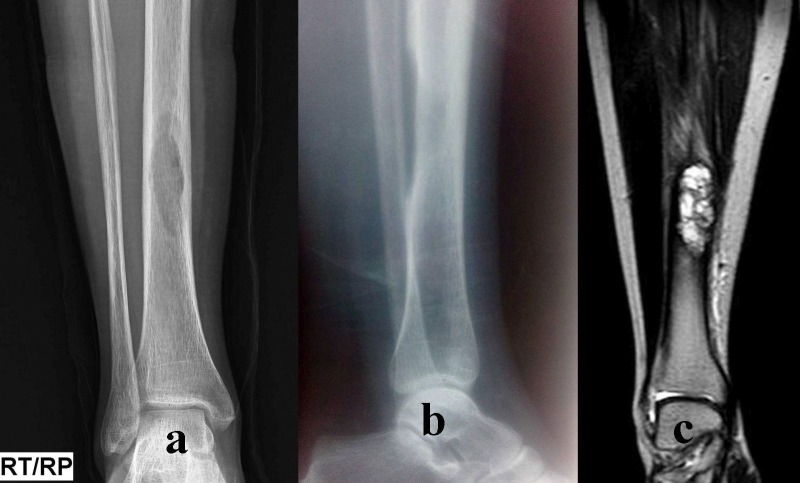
Figure 1(a) anteroposterior, 1(b) lateral views of brown tumor of right tibial diaphysis in 25-year-old female patient. 1(c) magnetic resonance imaging (MRI) showing well-defined expansile lytic lesion along the anterior medial aspect of the middle third of shaft of the right tibia. Hyperintense on T2 and iso to hypointense on T1.
